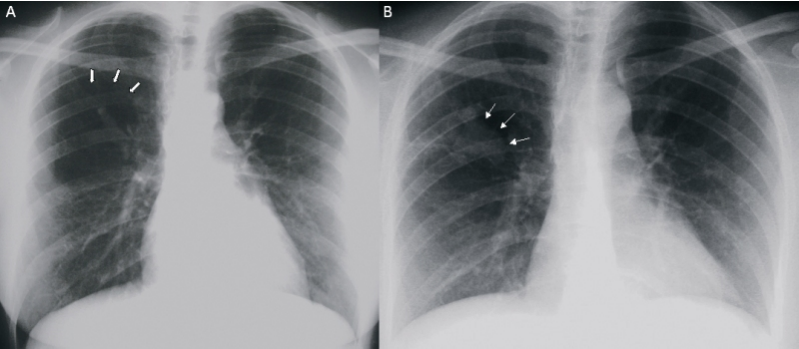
(a) Posteroanterior chest X-ray showing an area of over-inflation and parenchymal oligemia downstream at the right upper lobe (thick arrows). (b) Posteroanterior chest X-ray showing right parahilar superimposed opacity (thin arrows).
